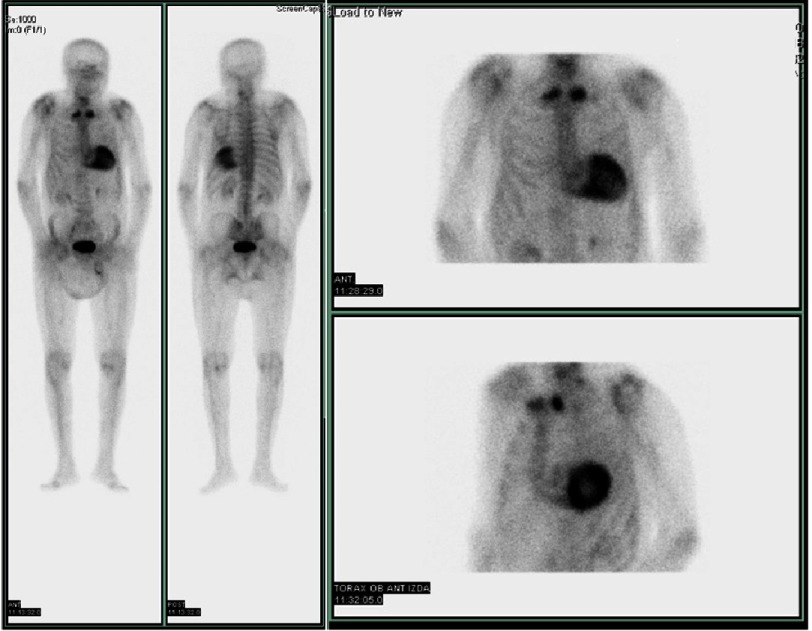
Bisphosphonate scintigraphy. Grade 3 uptake score in a patient with ATTR-CA.
